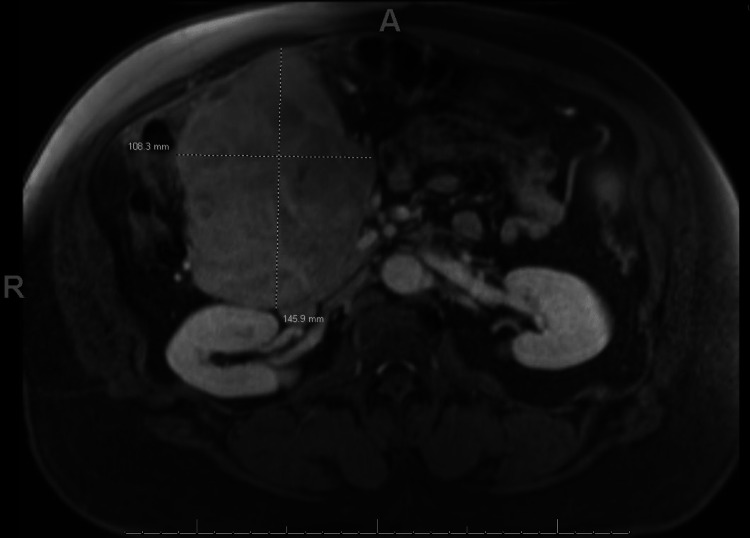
MRI abdomen/pelvis w/wo contrast showing a large heterogeneously enhancing mass in the second portion of the duodenum measuring 14.6 x 10.8 x 12.4 cm.
